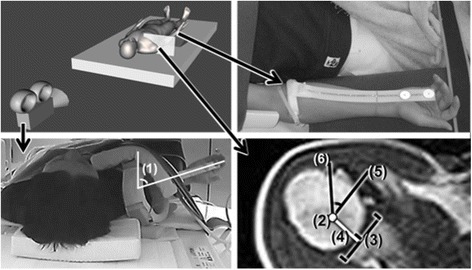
Experimental imaging. Fig. 1a (left top) shows the view of the video camera that was synchronized with the MRI. Fig. 1b (right top) shows a non-constraining brace applied to the subject’s forearm. The position of the brace was not affected by pronation or supination of the forearm. Fig. 1c (left bottom) is a frame captured by the video camera, showing (1) the shoulder rotation angle. Fig. 1d (right  bottom), taken from an MRI scan, shows how translation of the humeral head and rotation angle on MRI are defined. (2) Center of the humeral head. (3) Center of the glenoid fossa. (4) Line from the center of the humeral head perpendicular to the glenoid. Translation of the humeral head is defined as the distance between (3) and (4). (5) Line parallel to a line connecting the anterior and posterior edges of the glenoid and crossing the center of the humeral head. (6) Line connecting the bicipital groove to the center of the humeral head. The rotation angle of the humeral head is defined as the angle between (5) and (6)
